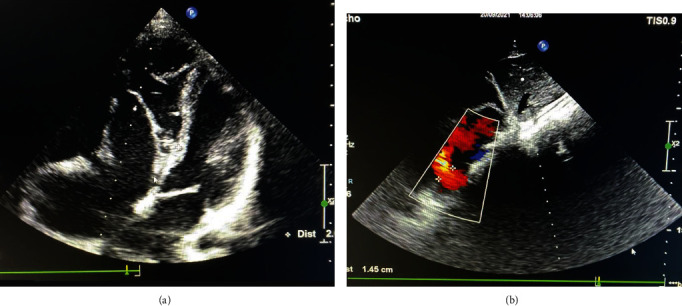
(a) Echocardiogram shows situs solitus, levocardia, left side aortic, and Ebstein types B-C. (b) ASD size of 8.3 mm with left to right shunt.
